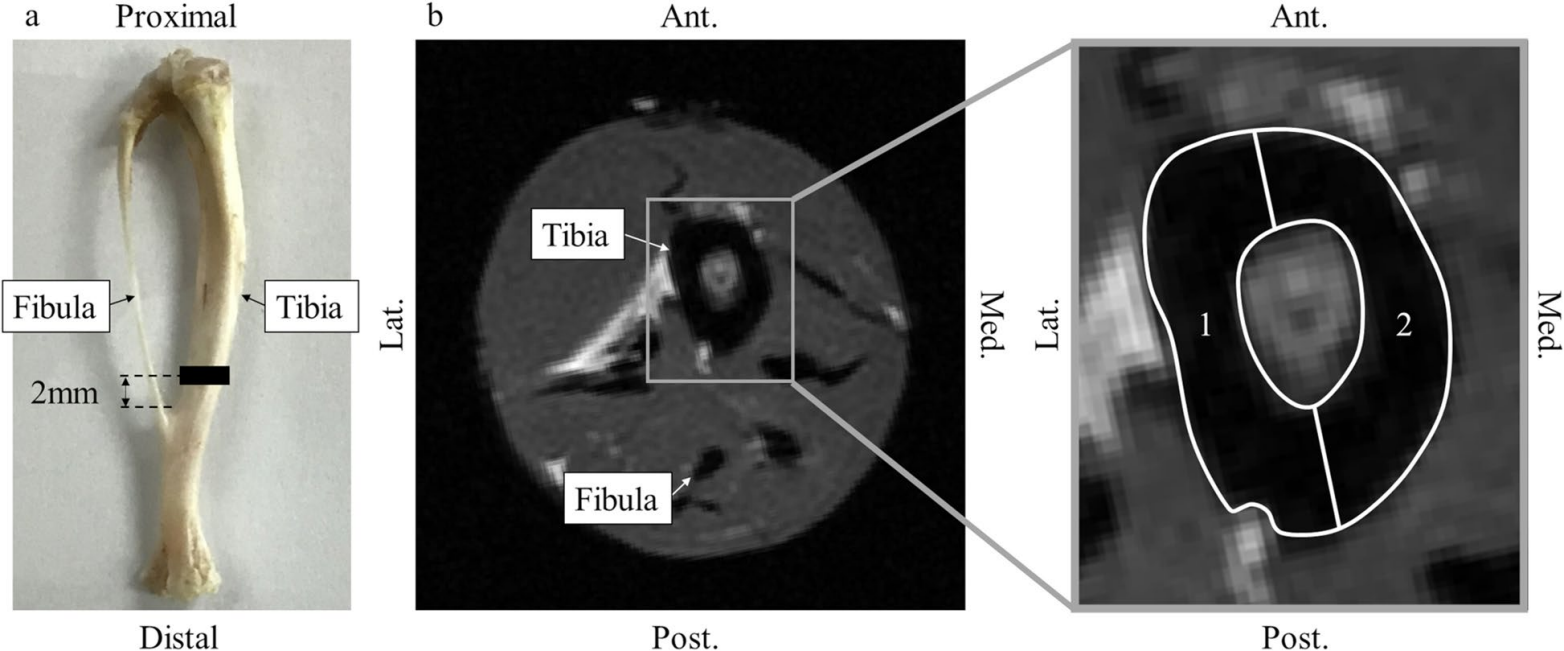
Regions of interest for MRI. a The regions are set 2 mm proximal to the tibiofibular union. b Two regions, including all the areas of cortical bone, are set in the cortical bone of the tibia in the axial plane (proton density-weighted image). Ant. = anterior, Post. = posterior, Med. = medial, Lat. = lateral
